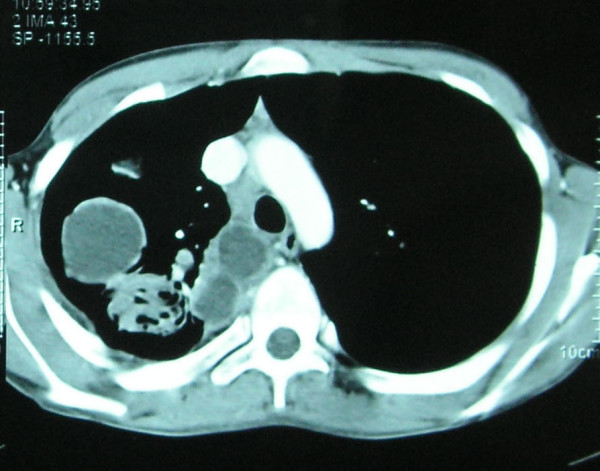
A cystic lesion 4 cm in diameter revealed by computed tomography in the posterior segment of the upper lobe of the right lung and multiple lesions neighboring the former, some of which were cystic and others solid (largest was 2 cm in diameter).
